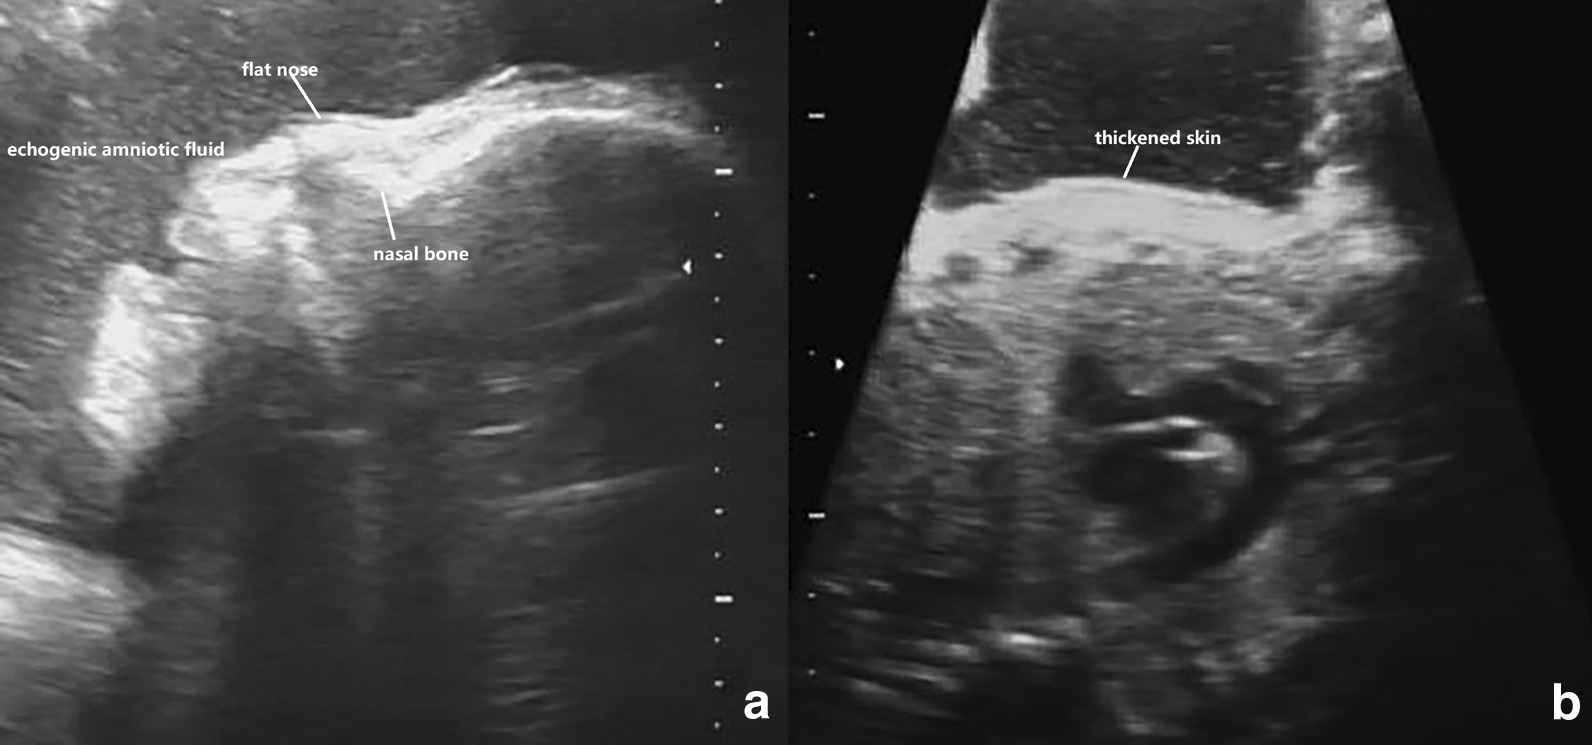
a 2-D ultrasound: flat nose and echogenic amniotic fluid at 31 weeks. b 2-D ultrasound: the long axis of the body shows locally thickened skin with a clear boundary with normal skin at 31 weeks
